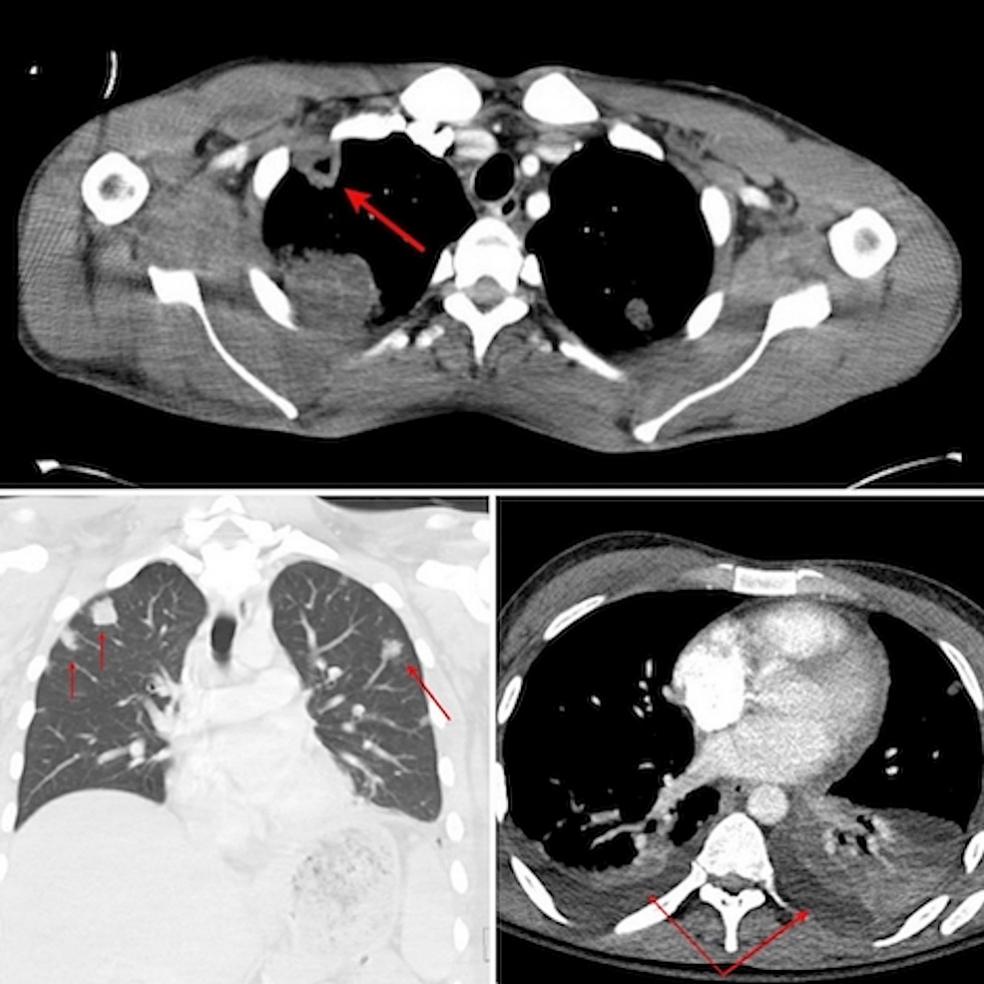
CT scan of the chest (red arrow) shows a cavitary lesion on top, bilateral septic (red arrow) pulmonary emboli (left side of the image), and bilateral pleural effusions (red arrow) more prominent on the left than the right side (right side of the image). CT: Computed tomography.
